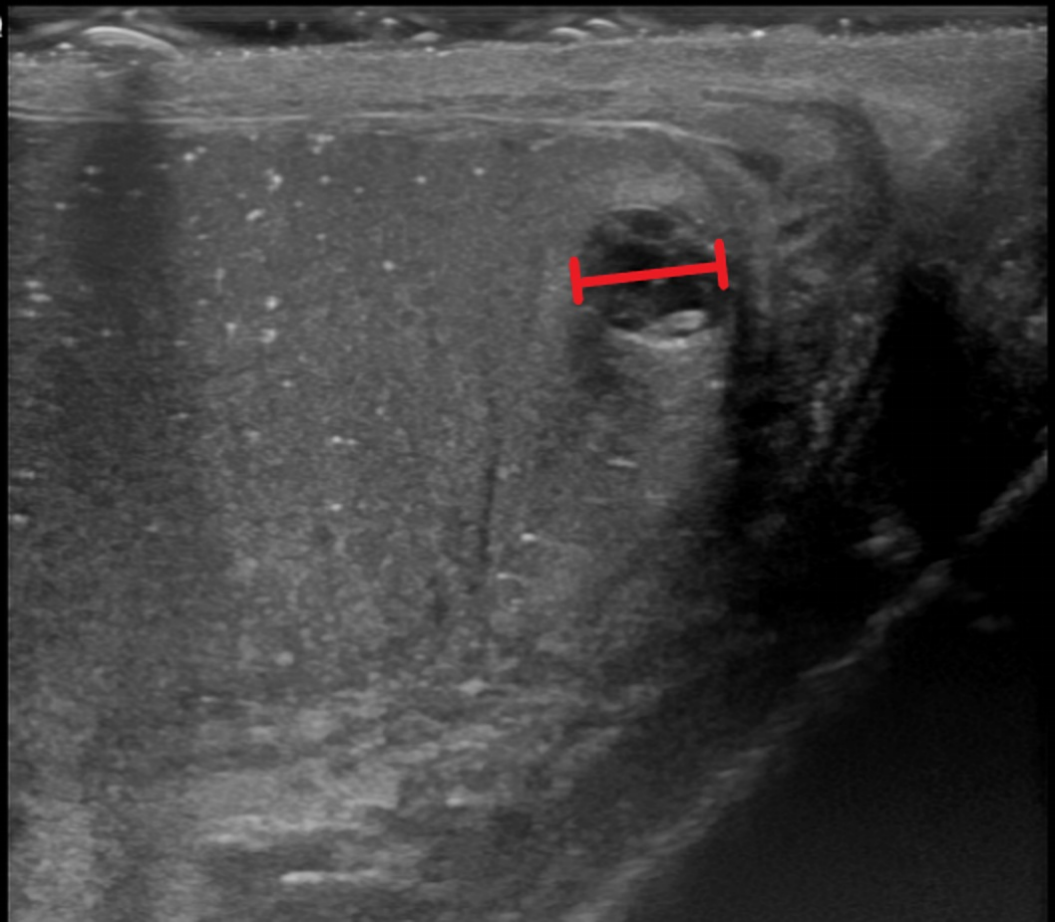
Ultrasound of the right testicle showing a complex multi-septated mixed lesion (red line)
